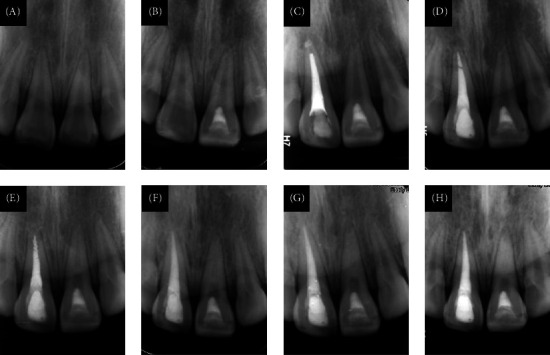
Tooth 21 of a 13-year-old boy diagnosed as a complicated crown fracture combined with subluxation and mature root formation that underwent full pulpotomy. (A) The preoperative periapical radiograph. (B–C) The 3- and 4-month follow-up periapical radiographs. (D) The radiograph at the 7-month follow-up showed external root resorption and a suspicious periapical lesion of tooth 21. (E–H) The radiographs at the 20-, 26-, 32-, and 38-month follow-ups. Spontaneous healing of the periapical lesion was observed, despite worsening pulp canal calcification.
